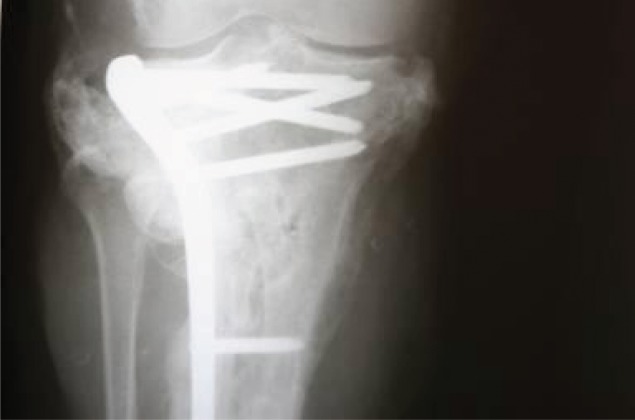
X-ray at 16 months; complete bony union and hypertrophy of the rib is noted
